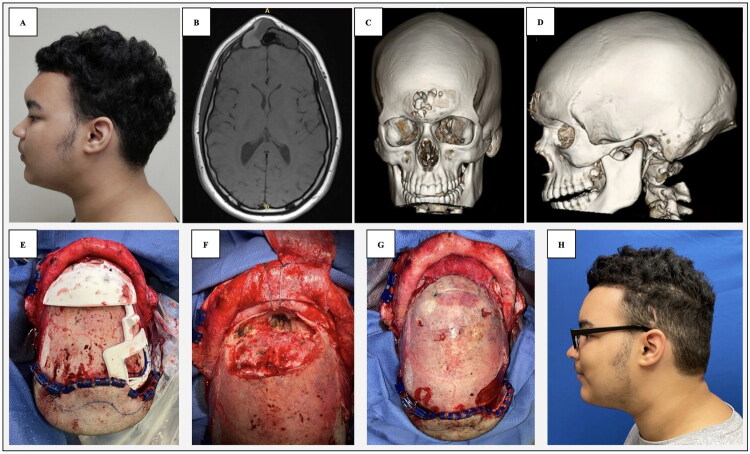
(A) Preoperative left profile of frontal sinus osteoma. (B) Preoperative magnetic resonance imaging with contrast, axial view. (C) Preoperative 3-dimensional computed tomography (CT) scan, frontal view. (D) Preoperative 3-dimensional CT scan, profile view. (E–G) Intraoperative frontal sinus reconstruction with split-calvarial bone graft and KLS Martin resorbable fixation system. (H) Postoperative left profile at 7-month follow-up.
